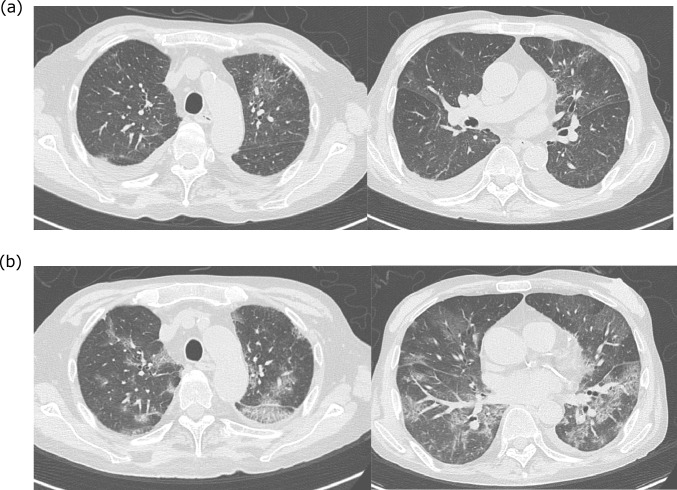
Findings from chest computed tomography (a) on hospital day 4 and (b) on hospital day 10. Bilateral ground-glass opacity and reticular opacity progressed between hospital day 4 and day 10
